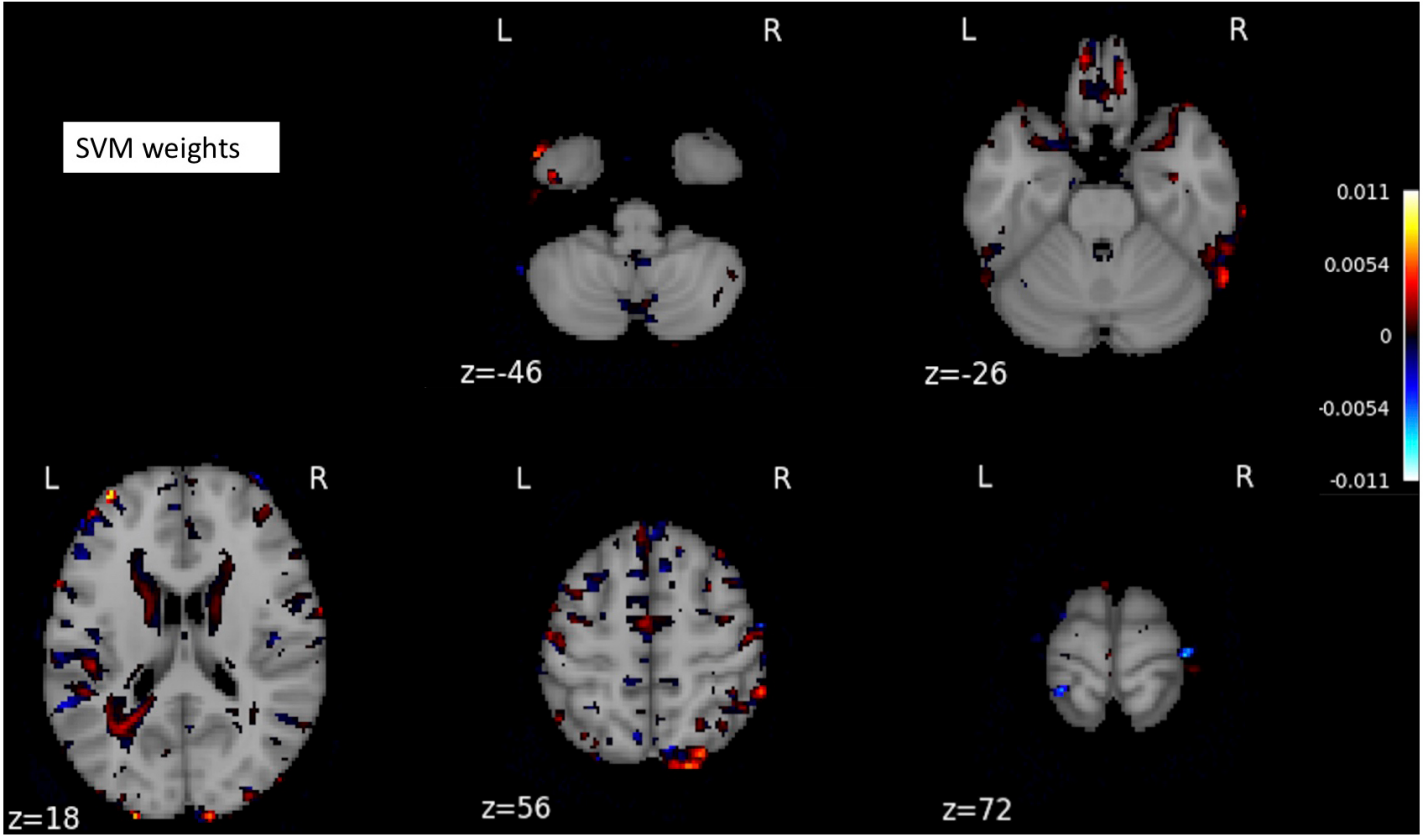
A structure scan in axial slices at different *z* levels. Projecting the weight vector back to an MR image yielded a map of the most discriminating regions between AD and CN (cognition normal). The highlighted red and blue regions represent the most discriminating regions in the original space, according to the SVM model learned from our dataset. The size of the MNI template used in visualization was 182 × 218 × 182.
